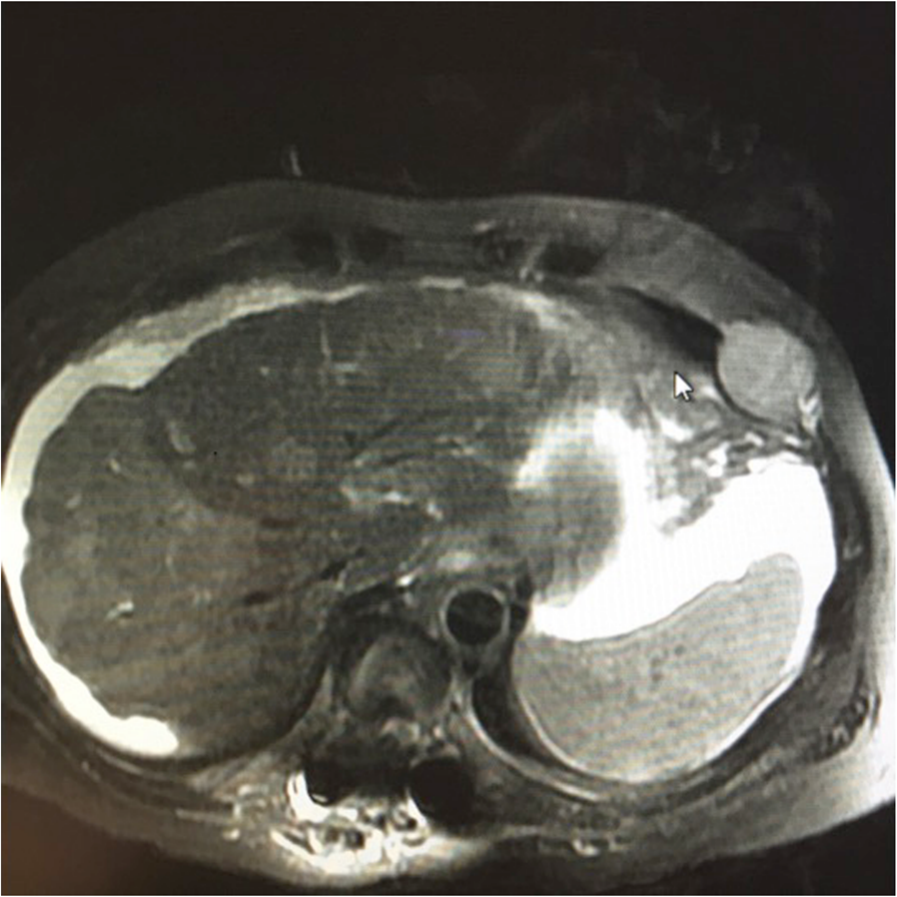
Magnetic resonance imaging of the thoracic spine. Axial T2 sequence demonstrates right chest wall and thoracic spine mass with tumor invasion into the spinal canal and thoracic cord compression at T6
